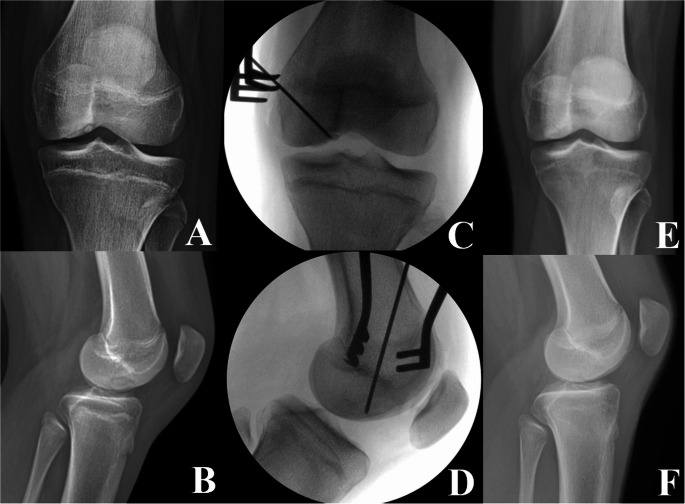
Retroarticular drilling for a stable medial femoral condyle lesion of a left knee. (A) and (B) demonstrate preoperative anteroposterior and lateral radiographs, respectively. (C) and (D) demonstrate intraoperative drilling using a start point below the physis. Anteroposterior and lateral radiographs at 9 months postoperative can be seen in (E) and (F), respectively
